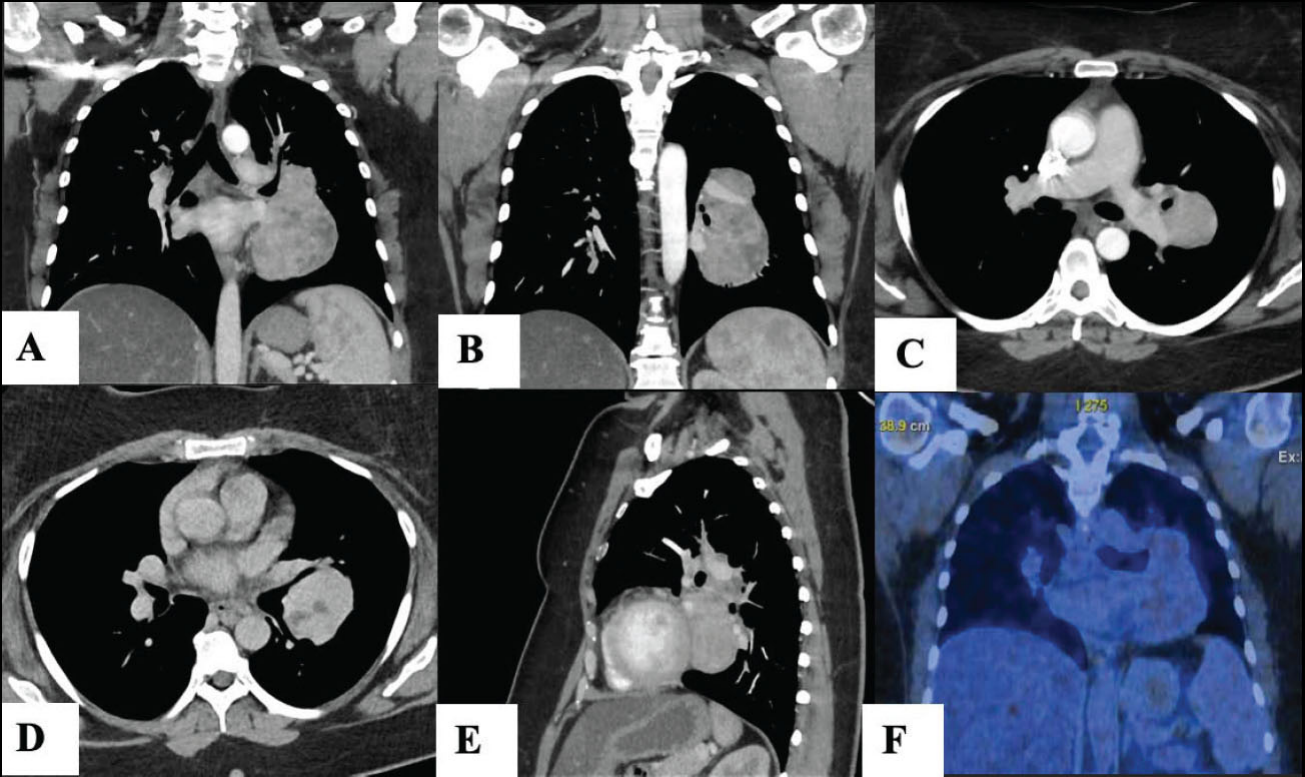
CT scan of the Unicentric Castleman tumor A) hemi-encircling the left upper lobe bronchus in coronal view, B) the inflow into the upper- and lower pulmonary vein in coronal view, C) the attachment and nutritive branch from the pars interlobaris of the pulmonary artery in axial view, D) the broad attachment in the bifurcation of the upper and lower lobe bronchus in axial view, E) the location with neighboring vessels and bronchi in sagittal view. F) PET-CT scan showing slight metabolic activity in the tumor.
